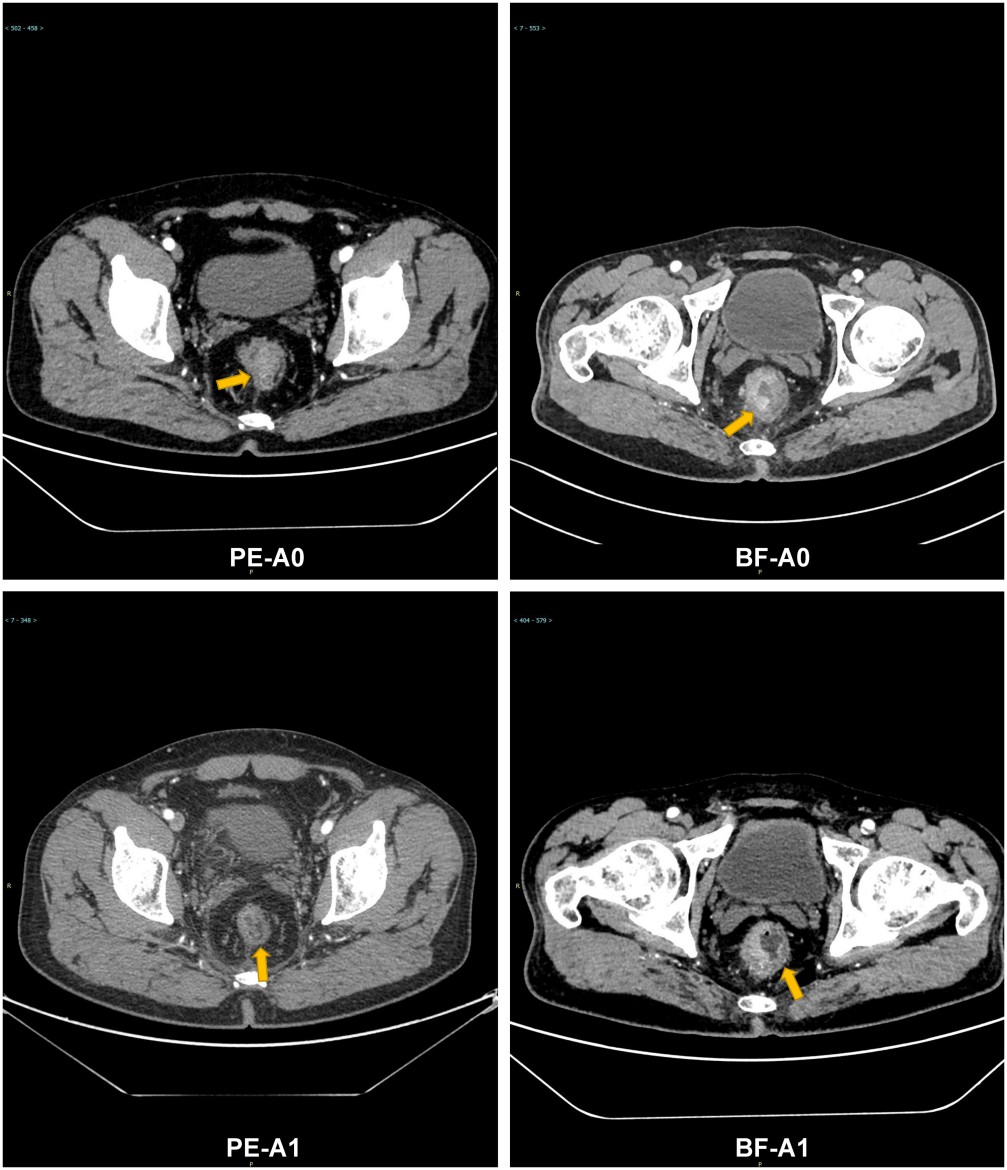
Comparative radiographic response of PE vs. BF in high TMB patients: tumor volume and Hounsfield unit calue changes at 6 months. Contrast-enhanced CT: PE-A0 (pre-treatment), PE-A1 (6-month post-PE); BF-A0 (pre-treatment), BF-A1 (6-month post-BF).
